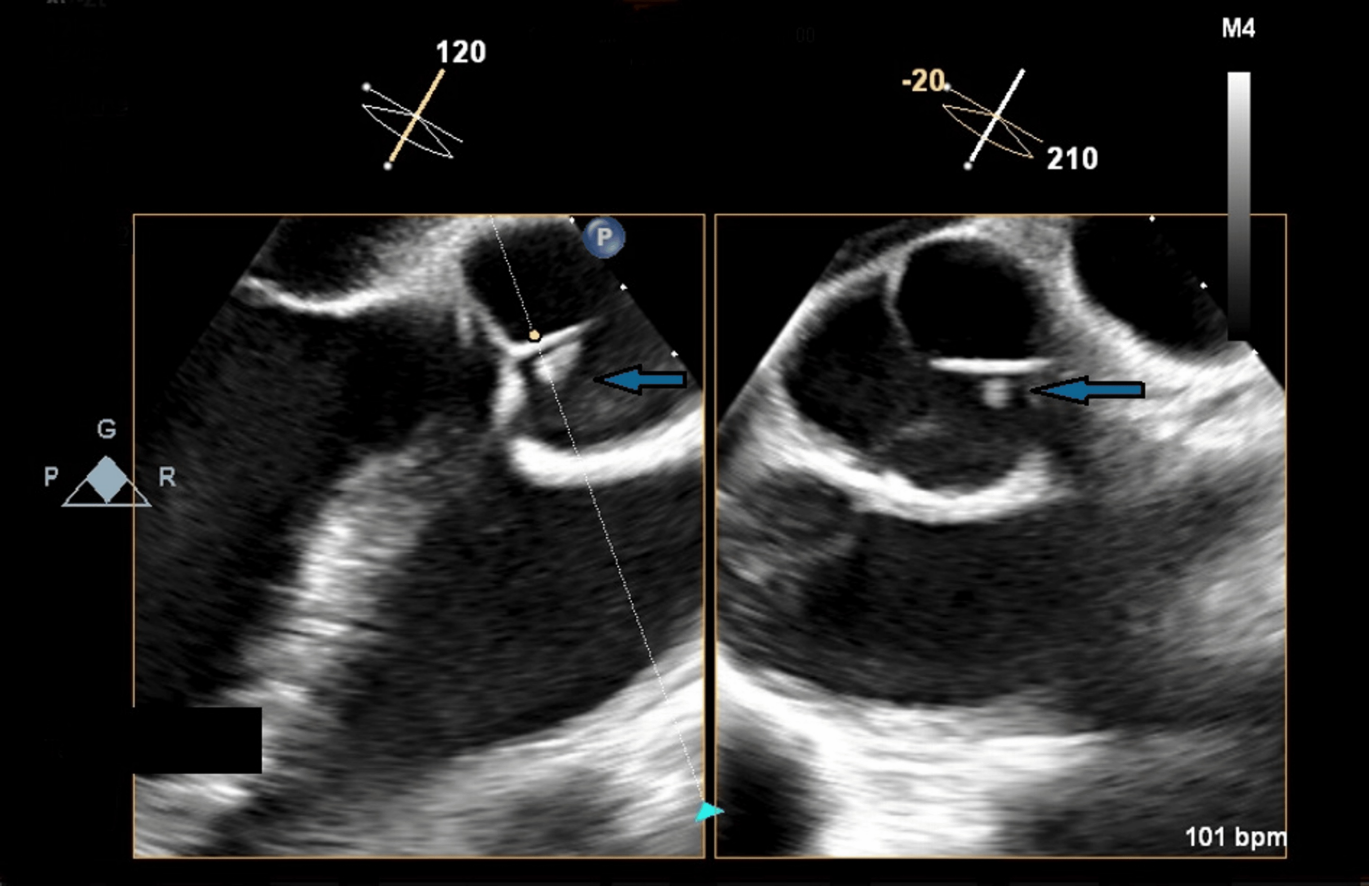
Transesophageal echocardiogram showing vegetation on the aortic valve.
